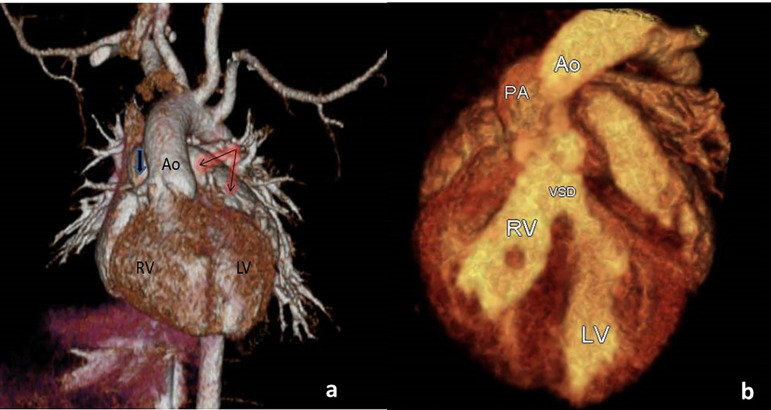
Three-dimensional computed tomography reconstruction image (a) representing the pulmonary trunk (bold arrow), aorta (Ao), and left juxtaposition of both atrial appendages (red shaded arrows); volume-rendering computed tomography image elaborating the anatomical relations in DORV (b). LV=left ventricle; PA=pulmonary artery; RV=right ventricle; VSD=ventricular septal defect
